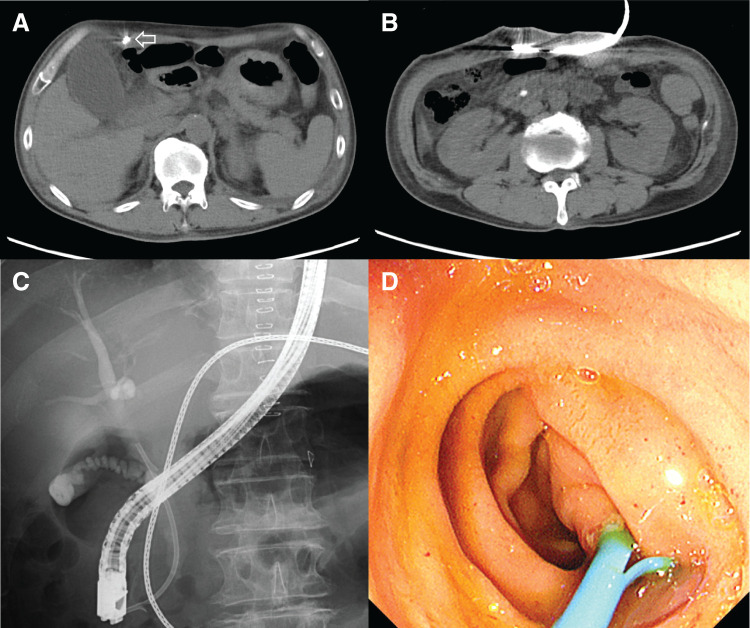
Preoperative imaging and endoscopic findings related to biliary tract infection. (A) Abdominal CT showed gallbladder wall thickening with a driveline running subcutaneously (arrow). (B) A stone is identified in the common bile duct. (C) Fluoroscopic image during endoscopic retrograde cholangiopancreatography confirmed placement of the ERBD tube. (D) Endoscopic image demonstrated placement of an ERBD tube through the papilla without sphincterotomy. ERBD, endoscopic retrograde biliary drainage
